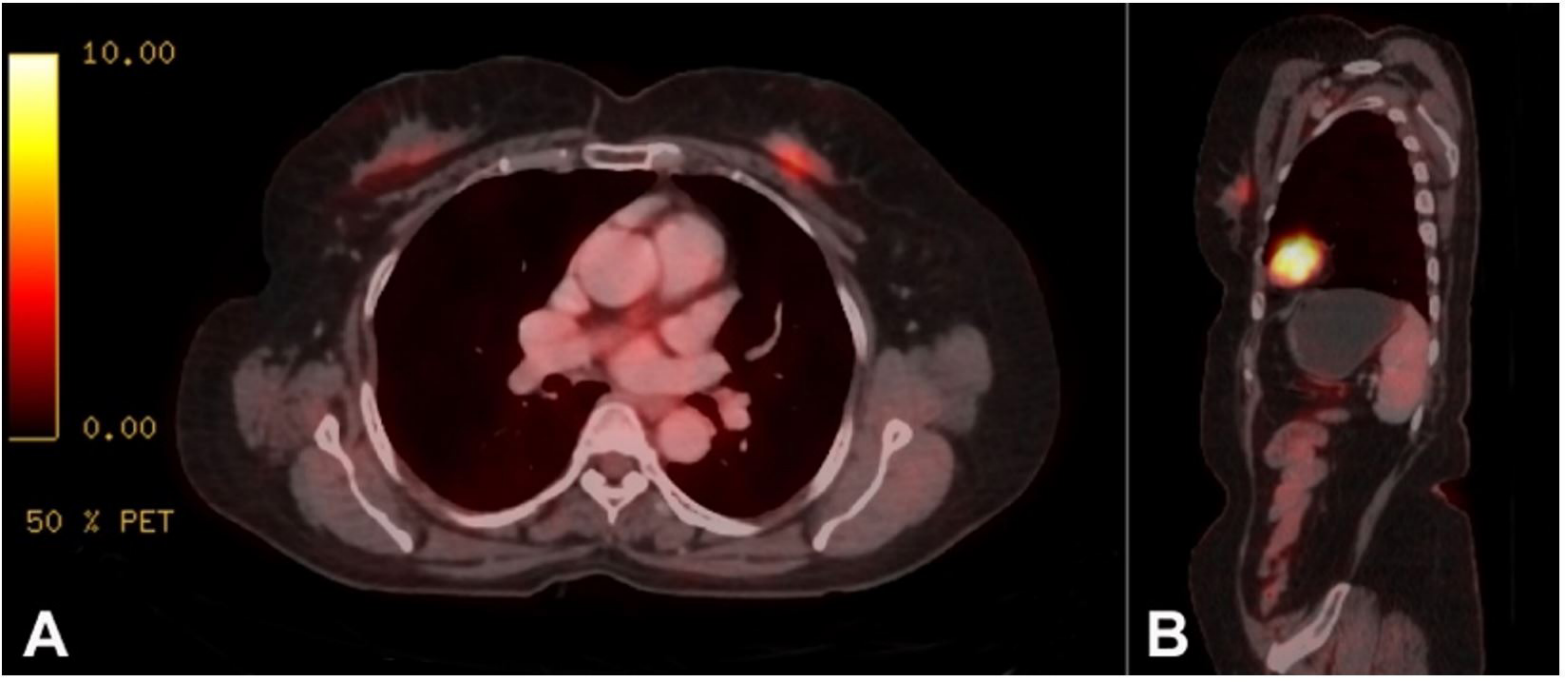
FDG-PET scan shows an ill-defined, hypermetabolic, heterogeneously enhancing FDG avid soft tissue nodular lesion involving upper inner quadrant of the left breast in axial view (Figure 1A) and parasagittal view (Figure 1B).
